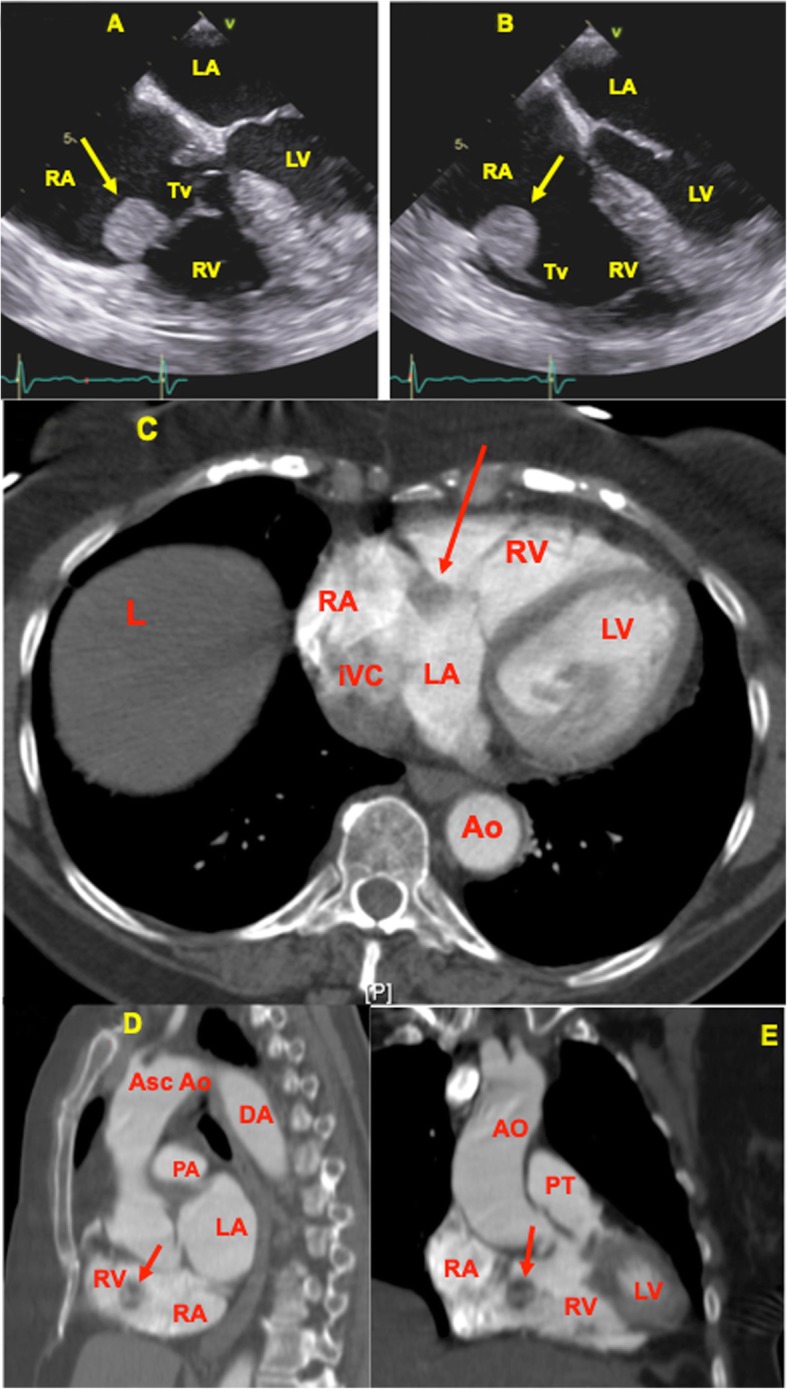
a: Transesophageal echocardiogram midesophageal four chamber view showing a 2.3 × 1.9 × 0.5 cm tricuspid mass (arrow), during systole. Abbreviations: RA-right atrium, RV-right ventricle, LA-left atrium, LV-left ventricle, Tv-tricuspid valve. b: Transesophageal echocardiogram midesophageal four chamber view showing partial obstruction of right ventricular inflow during diastole (arrow). Abbreviations: RA-right atrium, RV-right ventricle, LA-left atrium, LV-left ventricle, Tv- tricuspid valve. c: CT chest with contrast showing contrast enhanced axial CT scan with minimal enhancement of the mass (arrow) at the level of the tricuspid valve. The radiologist reported the finding as a 2.1 cm oval fatty mass seen along the tricuspid valve. Cardiac tumors are usually low attenuating. Abbreviations: RA-right atrium, RV-right ventricle, LA-left atrium, LV-left ventricle, Ao-aorta, IVC-inferior vena cava, L-liver. d: CT chest with contrast showing contrast enhanced sagittal CT scan with minimal enhancement of the mass (arrow). Cardiac tumors are usually low attenuating. Abbreviations: RA-right atrium, RV-right ventricle, LA-left atrium, Asc Ao-ascending aorta, PA-pulmonary artery, DA-descending aorta. e: CT chest with contrast showing contrast enhanced coronal CT scan with minimal enhancement of the mass (arrow). Cardiac tumors are usually low attenuating. Abbreviations: RA-right atrium, RV-right ventricle, LA-left atrium, LV-left ventricle, PT-pulmonary trunk, AO-ascending aorta
